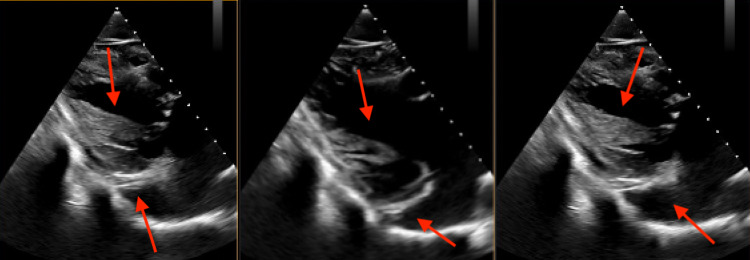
Transesophageal echocardiogram of the patient’s mitral valve Given the doming of the mitral valve, shortened chordae, and restricted papillary muscles as marked by the red arrows, these findings are all consistent withhammock mitral valve (mitral arcade)
